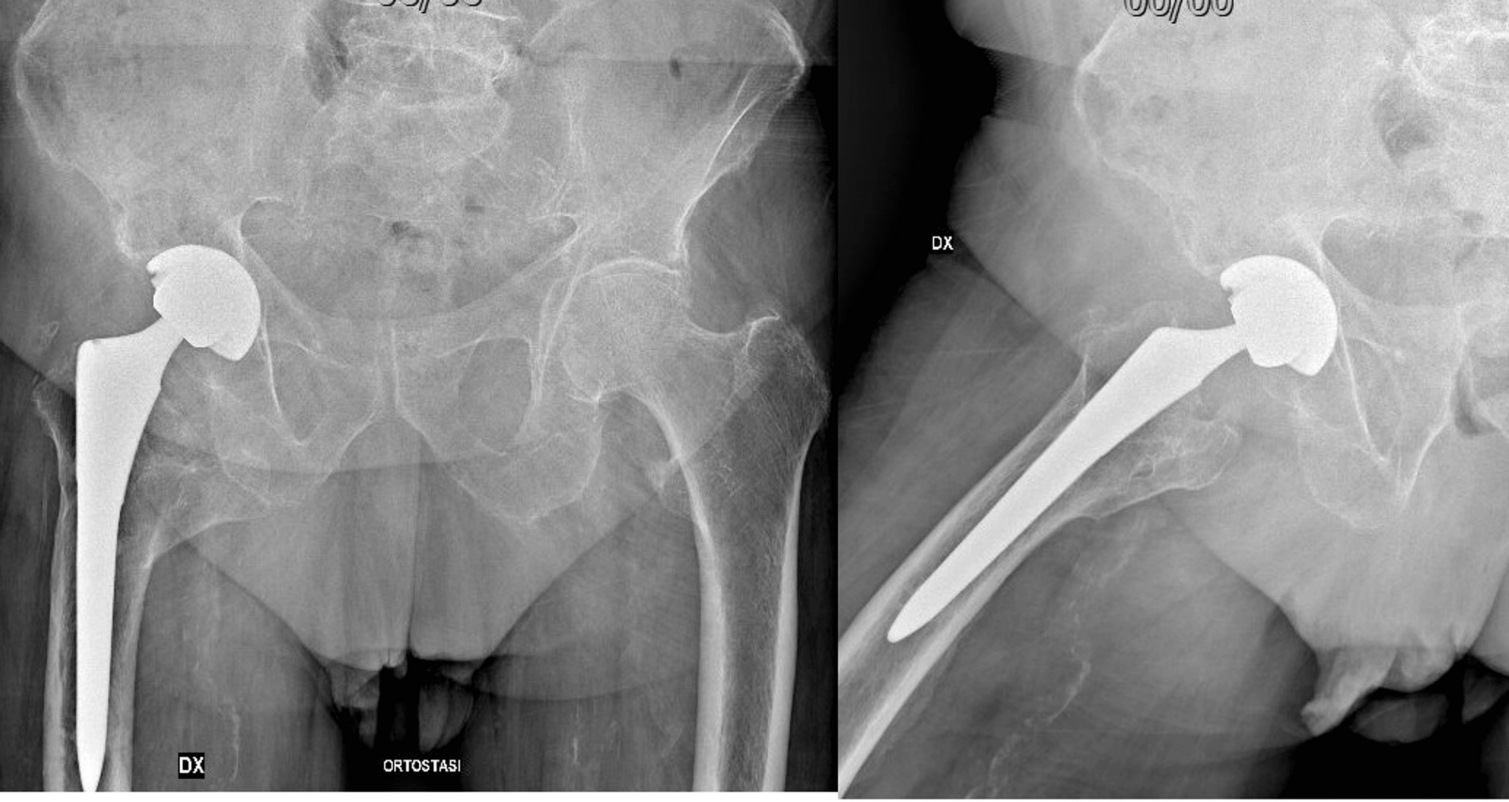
Anteroposterior radiographs of the pelvis and operated hip at the 10-year follow-up, showing stable cementless total hip arthroplasty with no evidence of loosening
